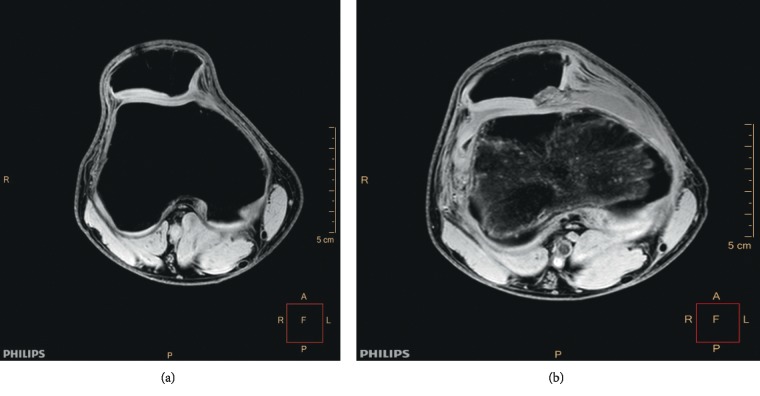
(a) Restored articular surface with irregular chondral healing in follow-up MRI WATS 3. (b) Preoperative MRI WATS 3D in the same patient.
